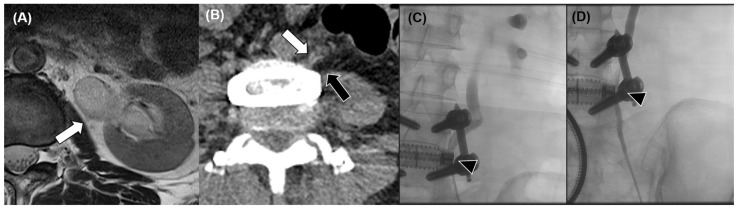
(A) Postoperative lumbar MRI demonstrating incidental dilatation of the left renal pelvis and proximal ureter (white arrow). (B) Contrast-enhanced CT showing abrupt angulation of the left proximal ureter toward the interbody cage at the L4 level (white arrow), adjacent to retroperitoneal soft tissue (black arrow). (C) Intravenous pyelography demonstrating focal narrowing of the ureter at the level of the interbody cage (black arrowhead). (D) Follow-up imaging showing improved ureteral narrowing (black arrowhead) with mild residual stenosis.
